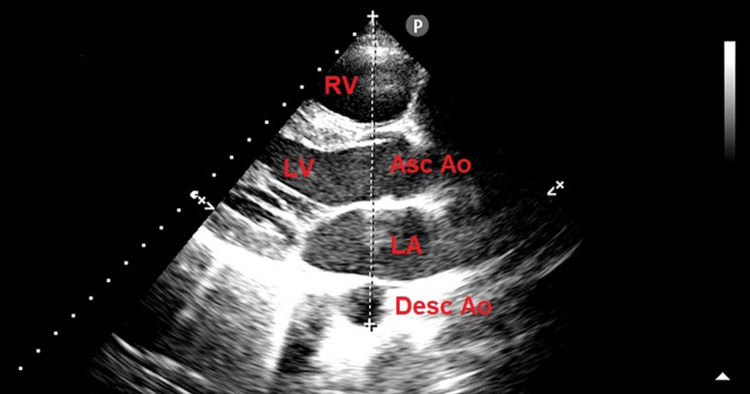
Echocardiographic assessment of the anteroposterior thoracic diameter. Transthoracic echocardiography, parasternal long-axis view: The white dotted line indicates the anteroposterior thoracic diameter, measured as the distance between the true apex of the sector and the anterior surface of the vertebral body. The latter is identified by using, as a reference point, the posterior wall of the descending thoracic aorta, visualized behind the left atrium. Ao, aorta; Asc, ascending; Desc, descending; LA, left atrium; LV, left ventricle; RV, right ventricle
